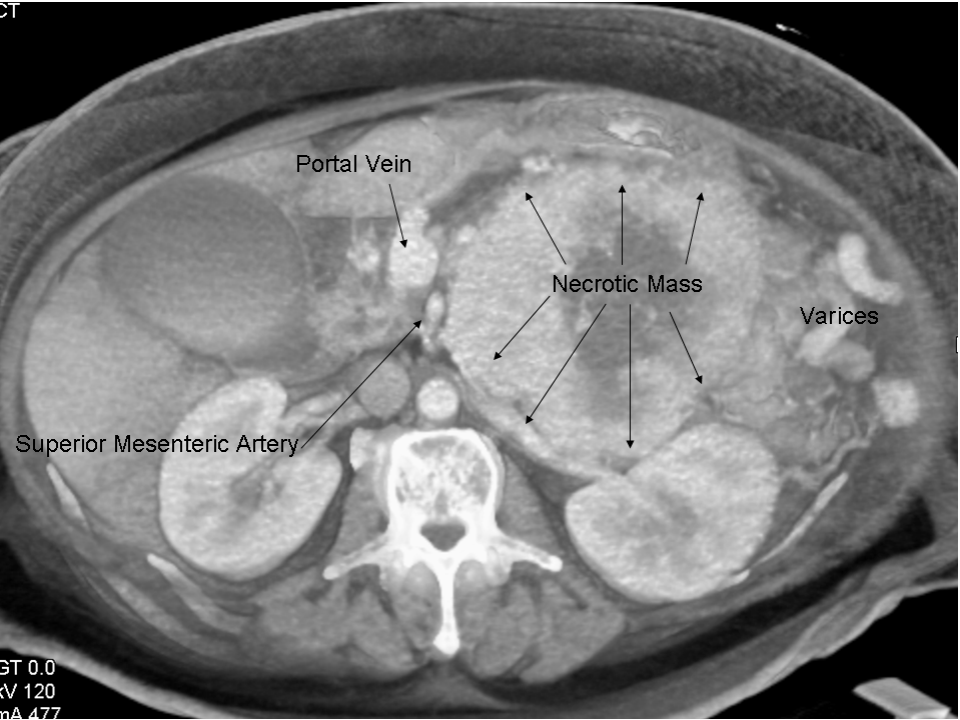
Cross-sectional CT depiction of large necrotic pancreatic VIPoma and its relation to the portal vein and superior mesenteric artery.
